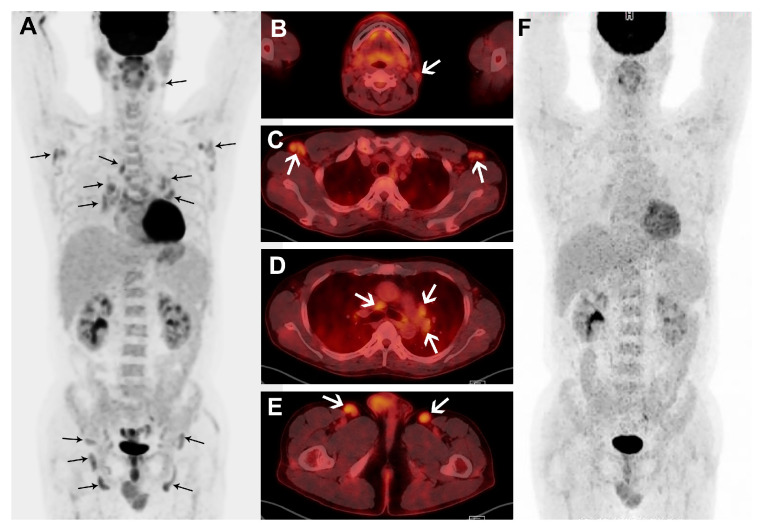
A male patient with plasma cell subtype of MCD confirmed by biopsy of right inguinal node. Maximum intensity projection (A) and transaxial fusion images (B–E) of treatment naïve FDG PET/CT show multiple, mildly enlarged LNs with mildly increased FDG uptake in bilateral cervical, axillary, mediastinal, bilateral hilar, perigastric, bilateral iliac, and inguinal areas (black and white arrows). The patient’s hemoglobin and albumin levels were low, while the hs-CRP level was high. In the FDG PET/CT performed to monitor the response to six cycles of chemotherapy, the nodal FDG uptakes are resolved in the maximum intensity projection image (F).
